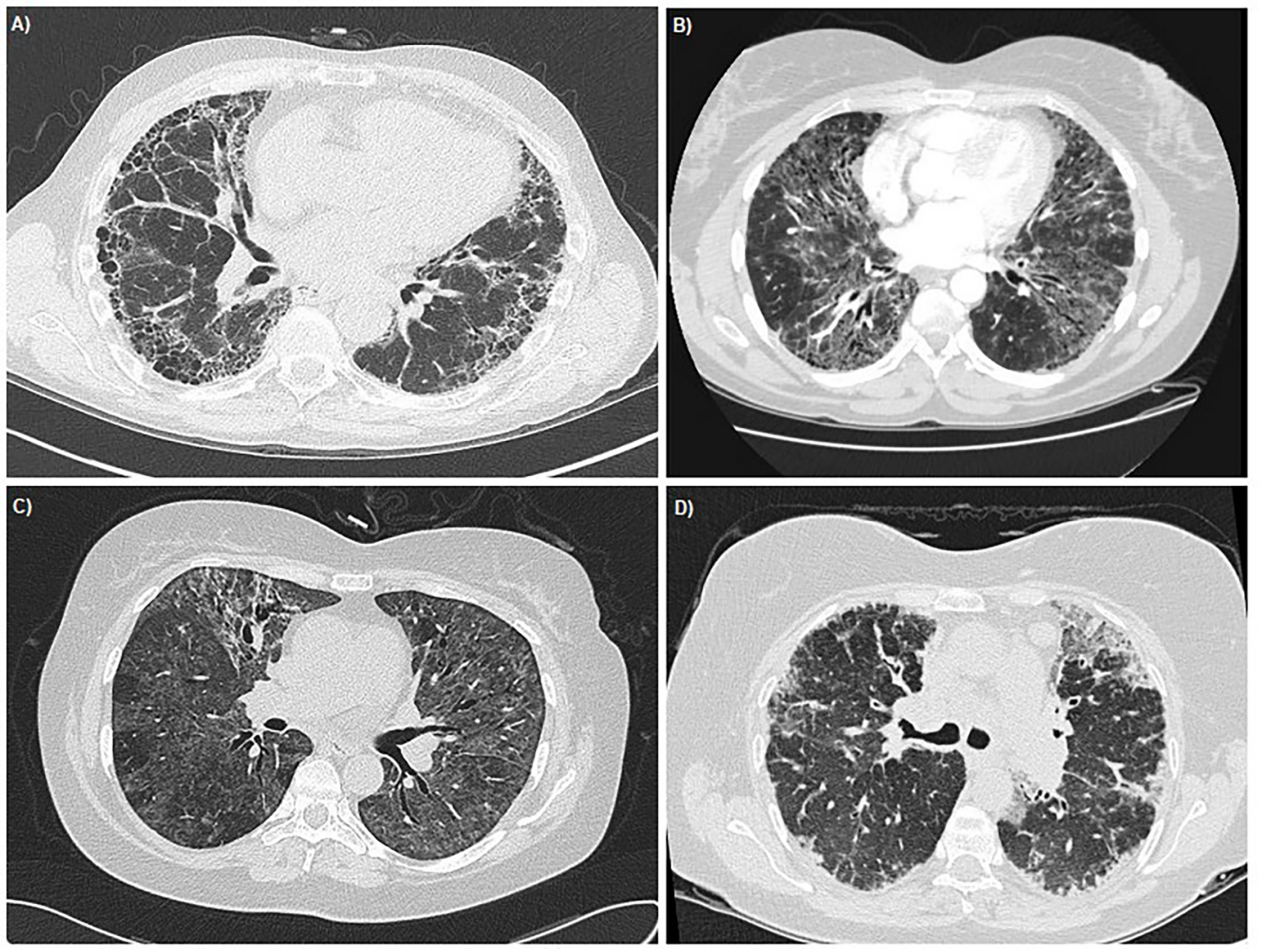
Thoracic HRCT images demonstrating the radiological appearances of the four sub-types of TIP. (A) Pulmonary Fibrosis - Case courtesy of Dr Ian Bickle, Radiopaedia.org, rID: 26493 (B) Acute Diffuse Interstitial Pneumonia - Case courtesy of Radswiki, Radiopaedia.org, rID: 11516 (C) Sub-acute Diffuse Interstitial Pneumonia - Case courtesy of Dr Mark Holland, Radiopaedia.org, rID: 19551 (D) Pulmonary Opacities with peripheral eosinophillia - Case courtesy of Dr Lawrence Josey, Radiopaedia.org, rID: 18019.
